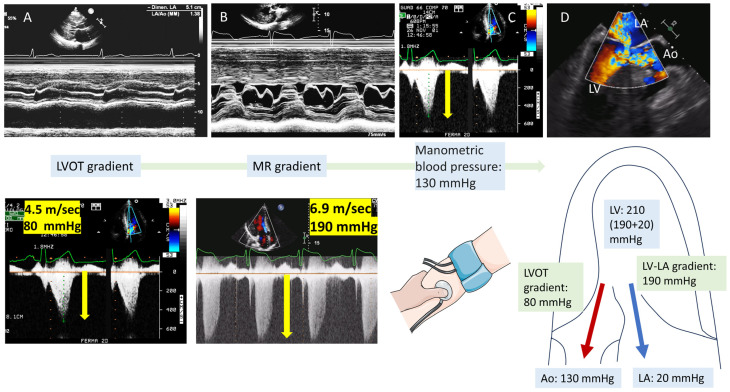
(A) Mid-systolic closure of the aortic box. (B) M-mode of the mitral valve with systolic anterior movement (SAM). (C) Left ventricular outflow tract (LVOT) obstruction with a typical dagger shape morphology. (D) Three-chamber long axis transesophageal echocardiography (TEE) view; the colour Doppler demonstrates the LVOT high velocity and the coexistence of mitral regurgitation (MR) due to SAM. Lower panels: how to calculate and validate the correct LVOT gradient from the MR gradient and manometric blood pressure (scheme of the simple test that may confirm the accuracy of the LVOT gradient measurement). Because the MR gradient reflects the difference between LV systolic pressure and left atrial pressure, it can be used to estimate LV systolic pressure when left atrial pressure is known or assumed. Simultaneously, the cuff-measured systolic blood pressure reflects aortic pressure, provided there is no significant aortic stenosis or LVOT obstruction. In HCM, the difference between the estimated LV systolic pressure and systolic blood pressure should equal the Doppler-derived LVOT gradient. If it does not, the LVOT gradient may be inaccurate, commonly underestimated due to poor Doppler alignment, or overestimated if the signal is contaminated by the MR jet. Ao: aorta.
